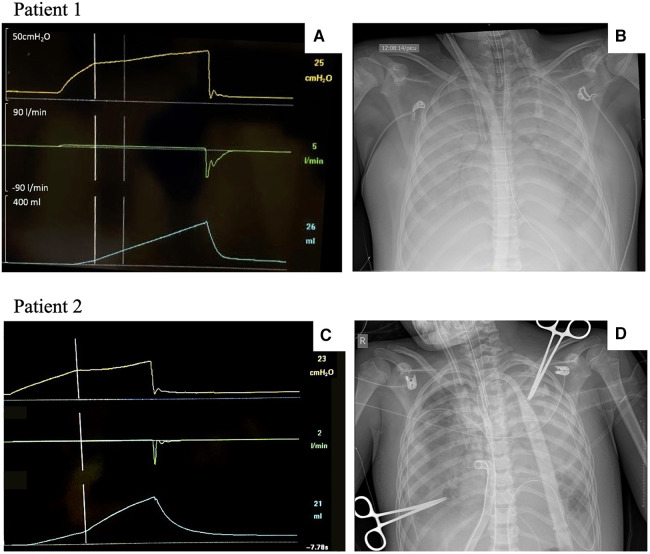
AOP maneuvers and chest x-rays in patients with p-ARDS. AOP, airway opening pressure; p-ARDS, pediatric acute respiratory distress syndrome. Patient 1: Adult AOP maneuver and chest x-ray in a 12-year-old patient with severe p-ARDS (A,B). (A) AOP maneuver with a clear identification of complete airway closure and an AOP at 25 cmH_2_O on Day 1 after ECMO cannulation. (B) Chest x-ray following cannulation. Patient 2: Adapted AOP maneuver and chest x-ray in a 9-year-old patient with severe p-ARDS (C,D). (C) Adapted AOP maneuver showing airway closure and an AOP at 23 cmH_2_O on Day 3 after ECMO cannulation. (D) Chest x-ray on the same day of the maneuver.
